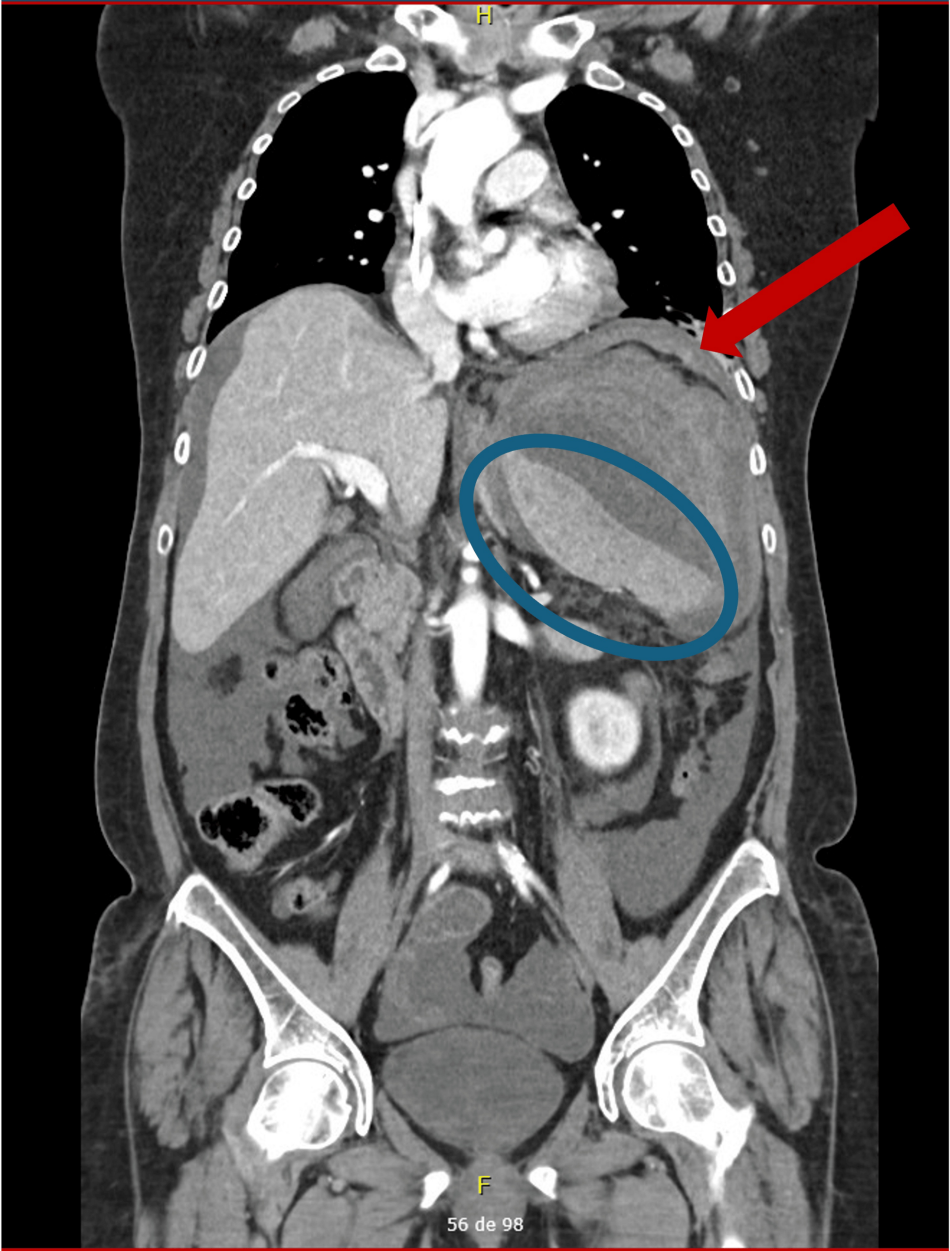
Coronal contrast-enhanced CT of the abdomen and pelvis blue circle: large subcapsular hematoma; red arrows: active contrast extravasation
